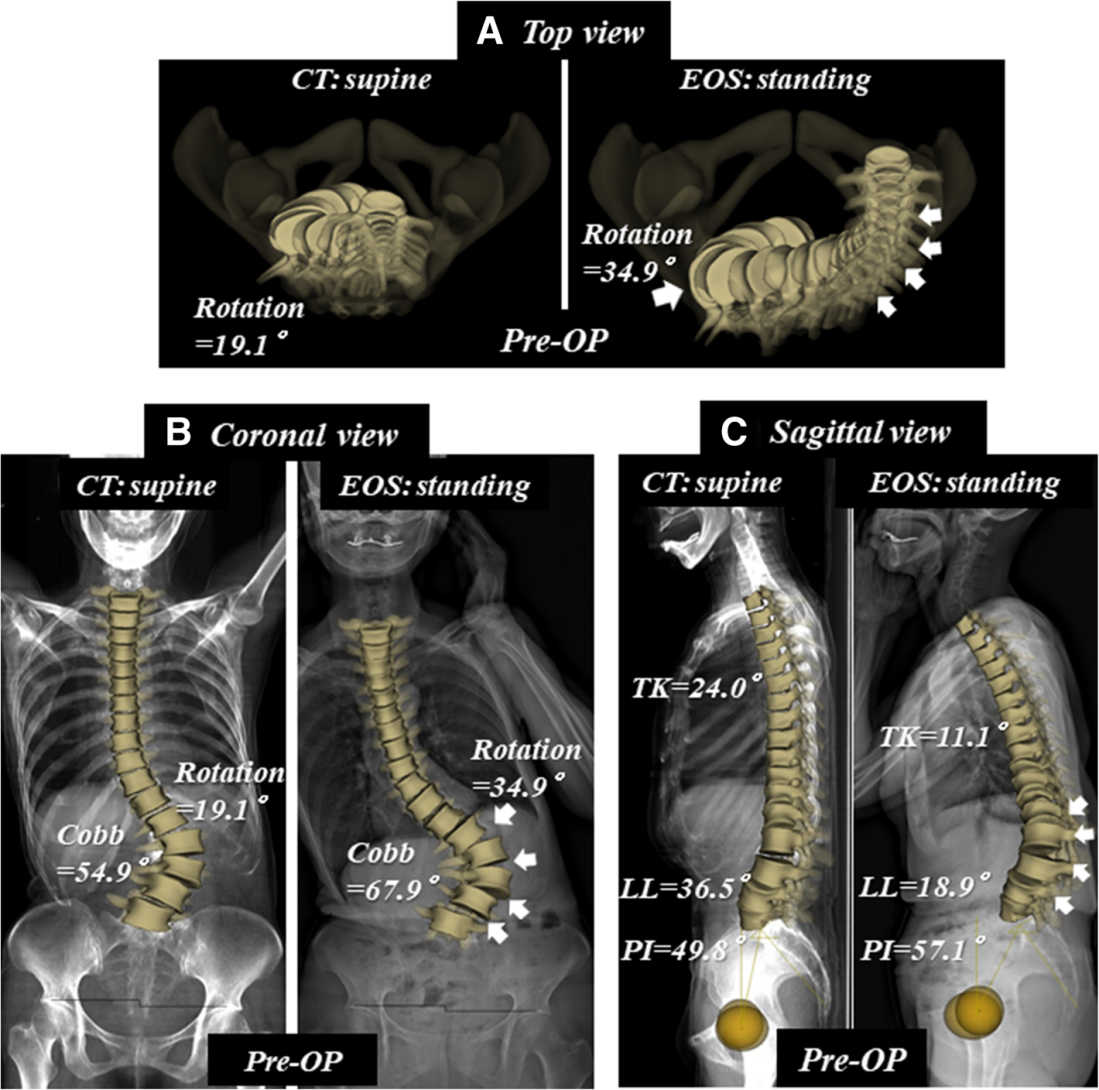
Patient with degenerative kyphoscoliosis. Preoperative supine CT and standing EOS images. a Top view images. Arrows show deterioration of T1 off-set and thoraco-lumbar rotation in standing position. b Coronal images. Arrows show deterioration of thoraco-lumbar curve in standing position. c Sagittal images. Arrows show deterioration of thoraco-lumbar kyphosis in standing position
